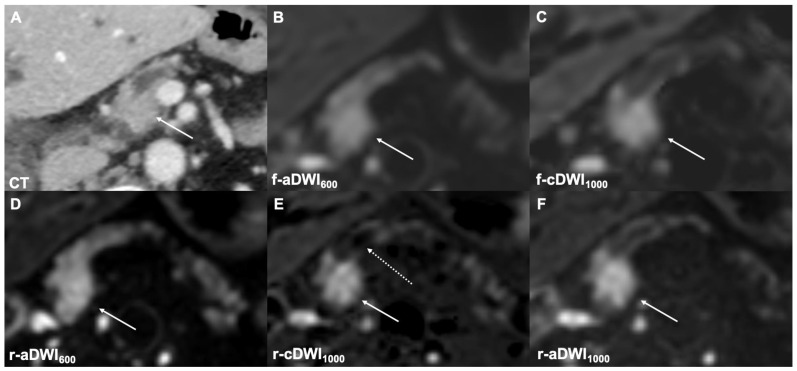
A CT image of a tumor in the pancreatic head causing obstruction of the main pancreatic duct (A). No clear tumor detection (type 3) is visible in the acquired images at b = 600 s/mm^2^ (B,D). The tumor conspicuity is superior in the images at b = 1000 s/mm^2^ (C,E,F). The fluid signal in the main pancreatic duct is best-suppressed in the r-cDWI1000 images ((E), dotted arrow). The labeling in image (E) has been corrected.
